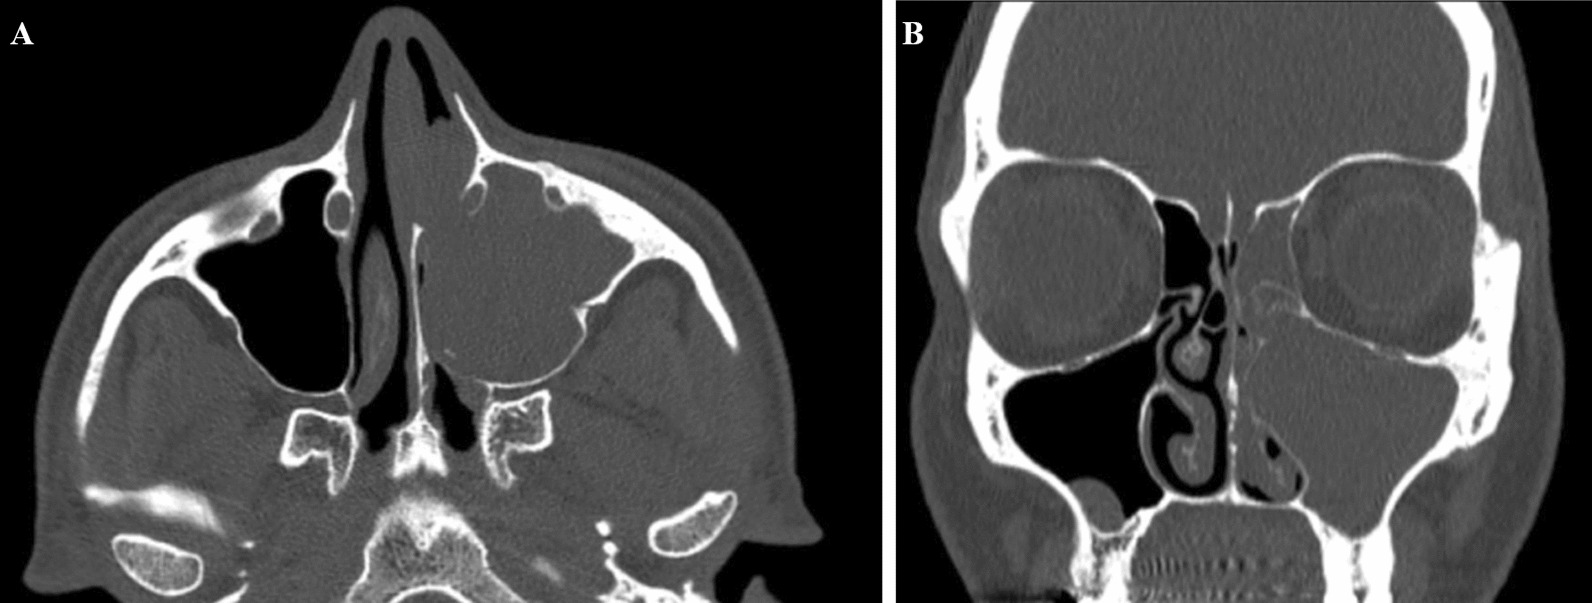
Head CT scan: a axial view, b coronal view. Evidence of a lesion, 24 mm × 28 mm × 26 mm in size, totally obliterating the left maxillary sinus, resulting in obstruction of the anterior osteomeatal unit and ethmoidal sinusitis
